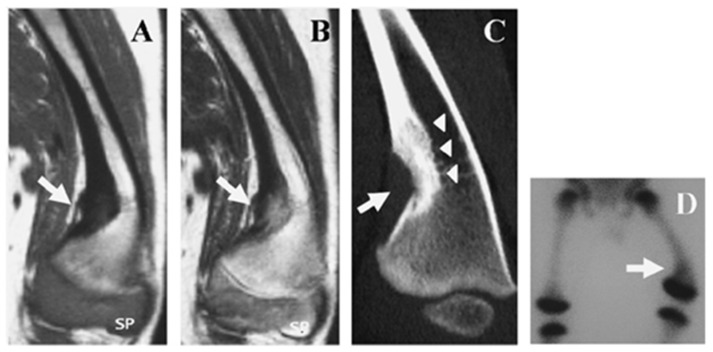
Imaging findings of FFCD in the distal left femur using different modalities. Reproduced from Ando et al., 2008 [60], with permission. Radiological findings of the lesion. (A,B) Magnetic resonance imaging showed lower signal intensities on T1-weighted images (arrow) and higher signal intensities on T2-weighted images (arrow) than muscles. An abnormal extraosseous mass was not apparent. (C) Computed tomography showed bony defect (arrow) with marginal sclerosis (arrowheads). (D) Bone scan with 99mTechnetium showed a mild uptake in the distal femur (arrow).
